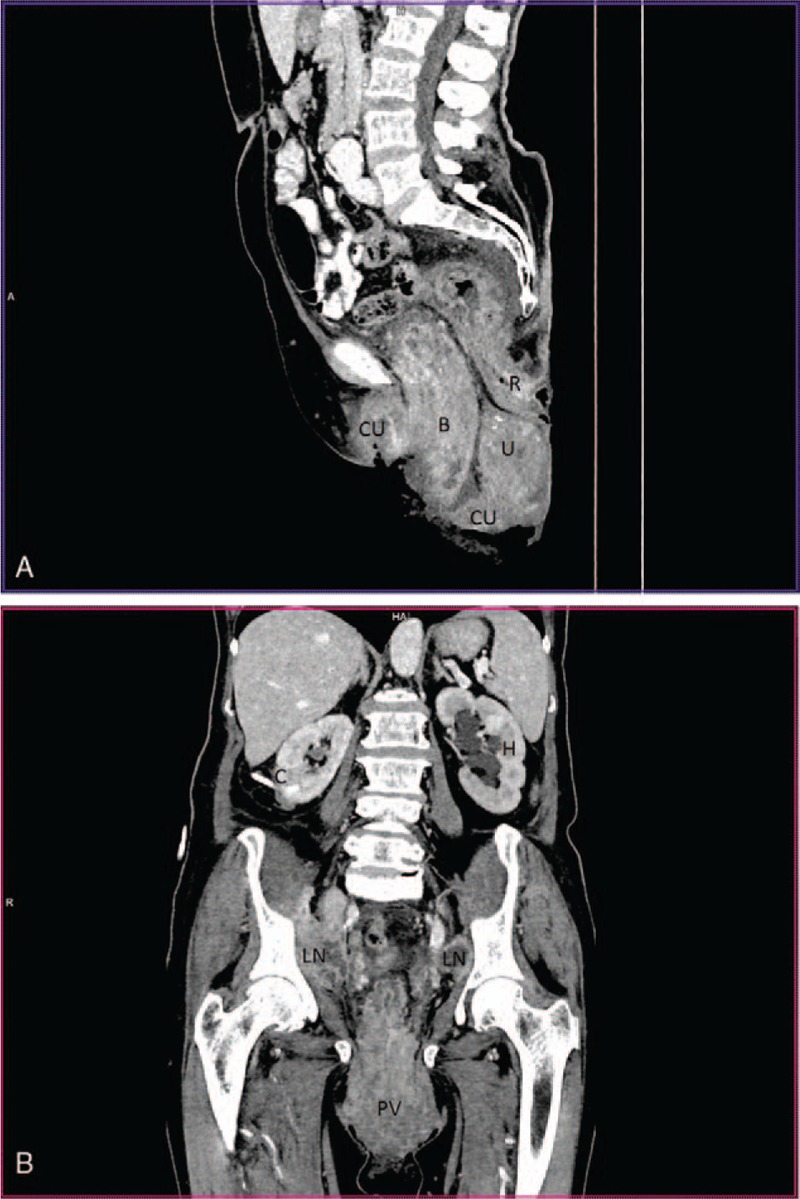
Sagittal pelvic computed tomography of the patient. In protruded vagina: bladder—B, rectum—R, carcinomatous ulceration—CU, uterus—U. Abdominal and pelvic frontal CT. PV—protruded vagina, LN—enlarged iliac nods, H—left kidney with hydronephrosis, C— nephrotomic catheter in right kidney.
